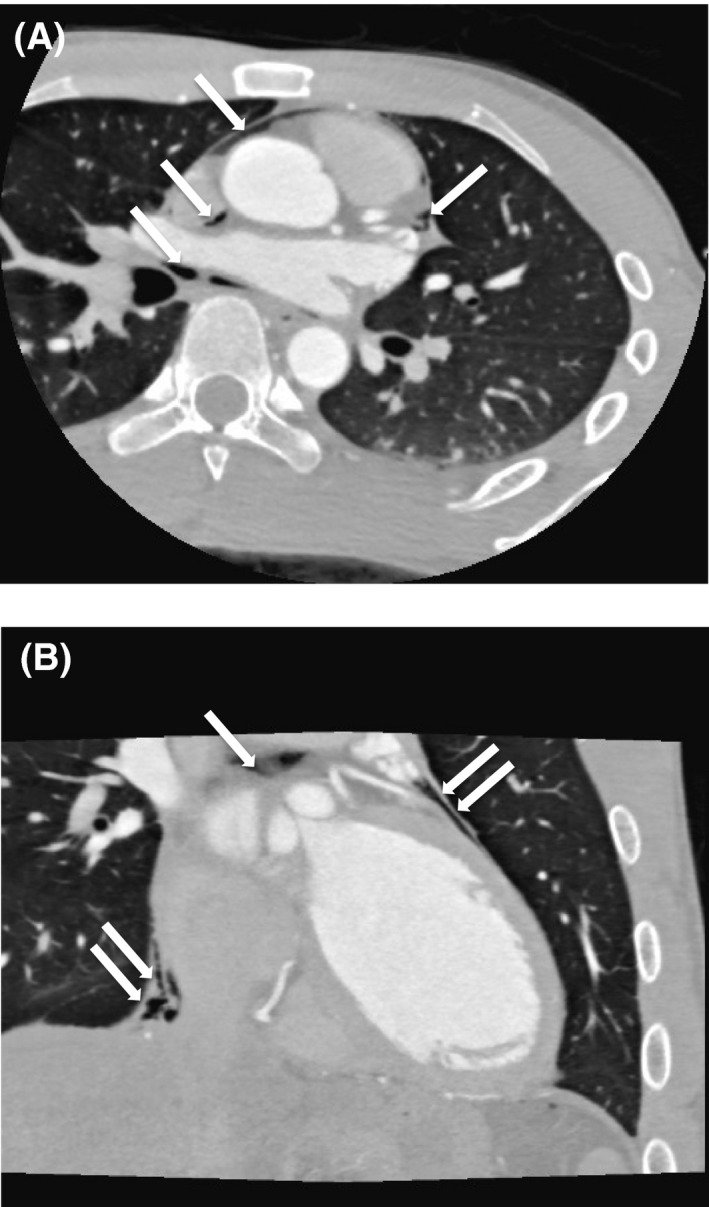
Computed tomography of the thorax: (A) coronal view and (B) sagittal view (arrows indicate air within mediastinum and pericardial space).
